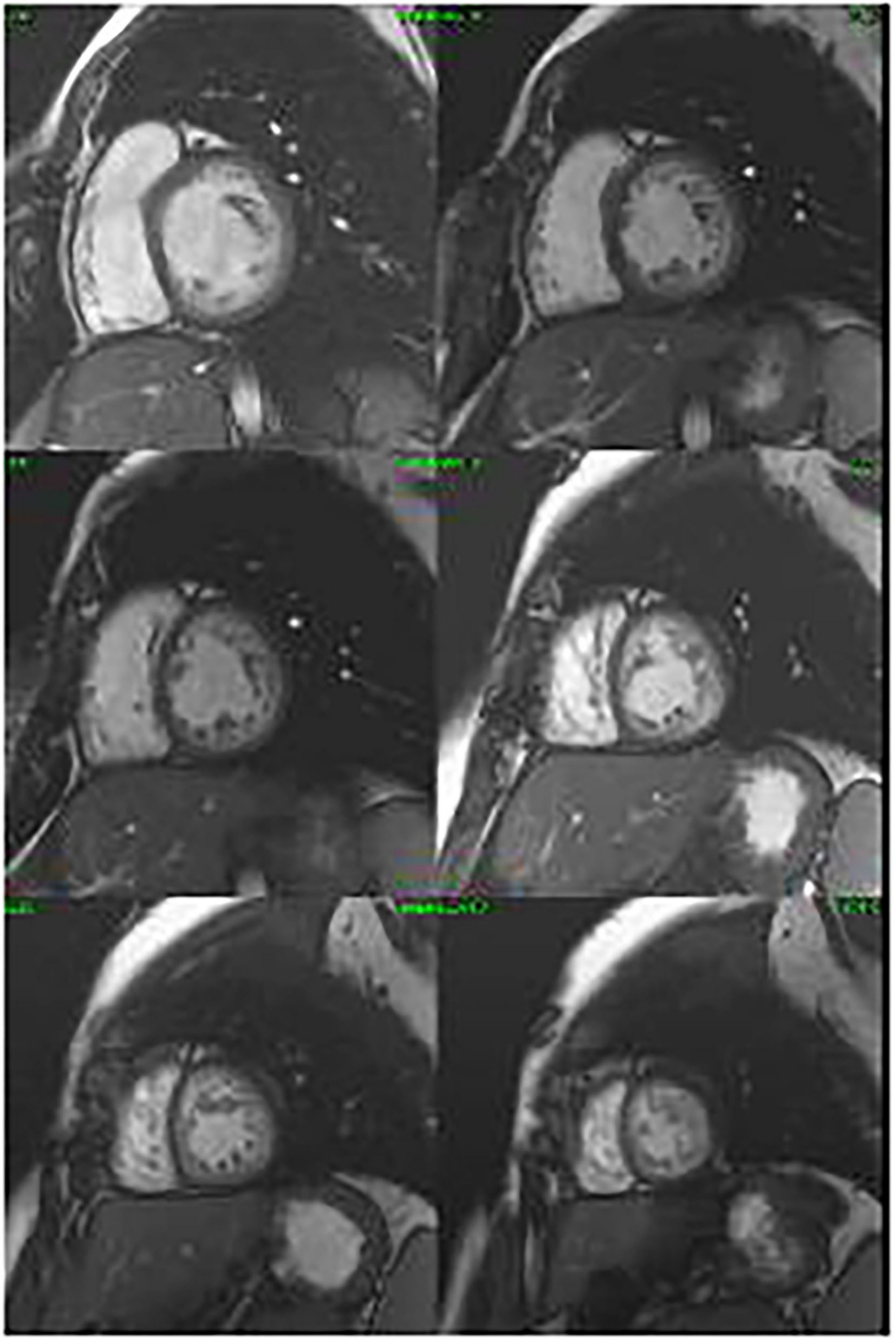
Cine cardiac magnetic resonance images in the short axis revealing non-compaction cardiomyopathy.
